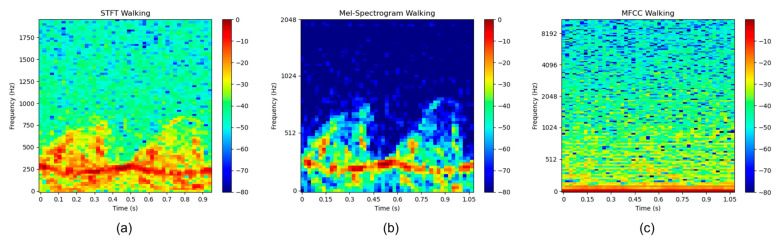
Micro-Doppler spectrogram of human walking in three time–frequency representation methods: (a) STFT, (b) Mel-spectrogram, and (c) MFCC.
